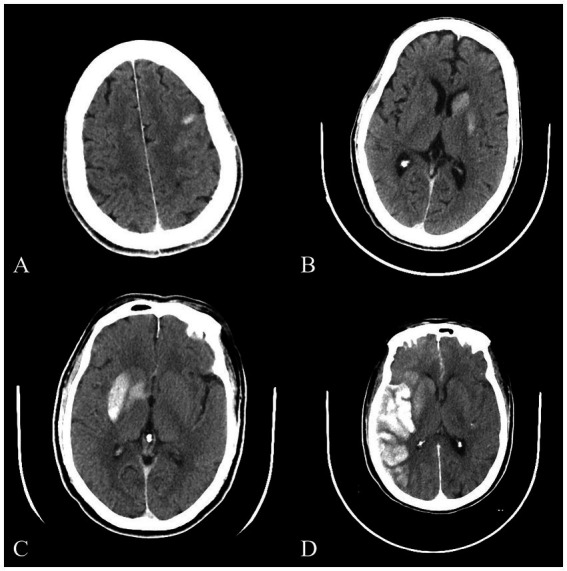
Typical images of four types of high-density shadow on immediate cerebral CT after successful interventional recanalization. (A) Cortical high-density shadow; (B) Soft high-density shadow; (C) Metal high-density shadow; (D) Diffuse high-density shadow. CT, computed tomography.
